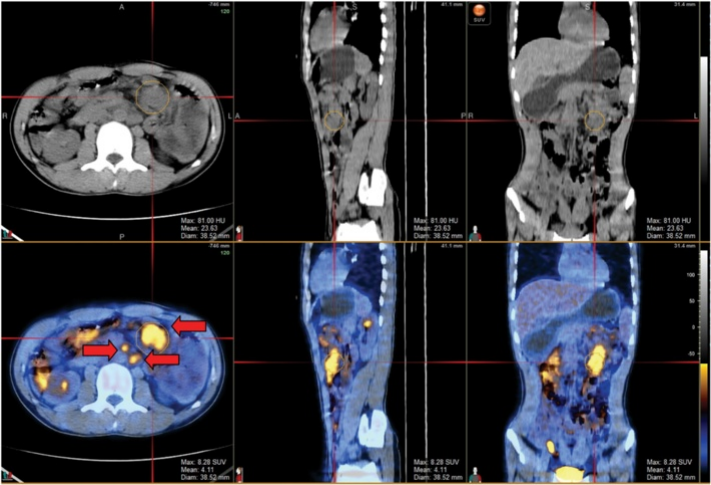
PET/CT scan image showing abnormal accumulations of ^18^F-FDP in many intestinal segments and also in many retroperitoneal swollen lymph nodes, indicating hypermetabolism disease, with a high possibility of a malignant disease
